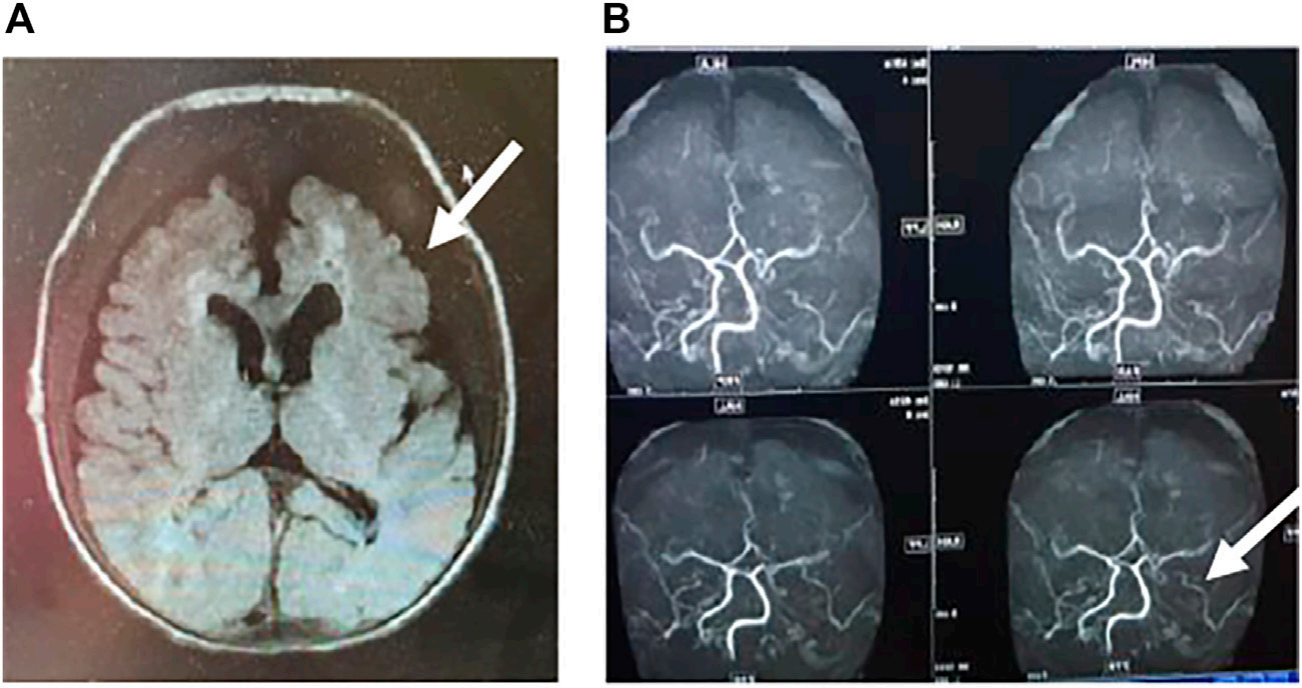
A) Cranial MRI showing bilateral frontal and temporal lobe atrophy and abnormally high signal in the white matter around the anterior horn of the ventricle. The lesions are indicated by arrows. (B) Cerebrovascular artery MRI showing multiple luminal stenoses of the bilateral anterior, middle, and posterior cerebral arteries and vertebral arteries. The lesions are indicated by arrows.
